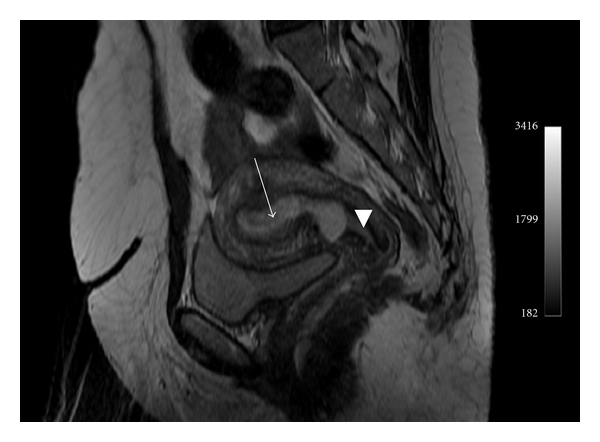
Magnetic resonance imaging cesarean scar pregnancy, empty uterus (arrow), empty cervical canal (arrow head).
